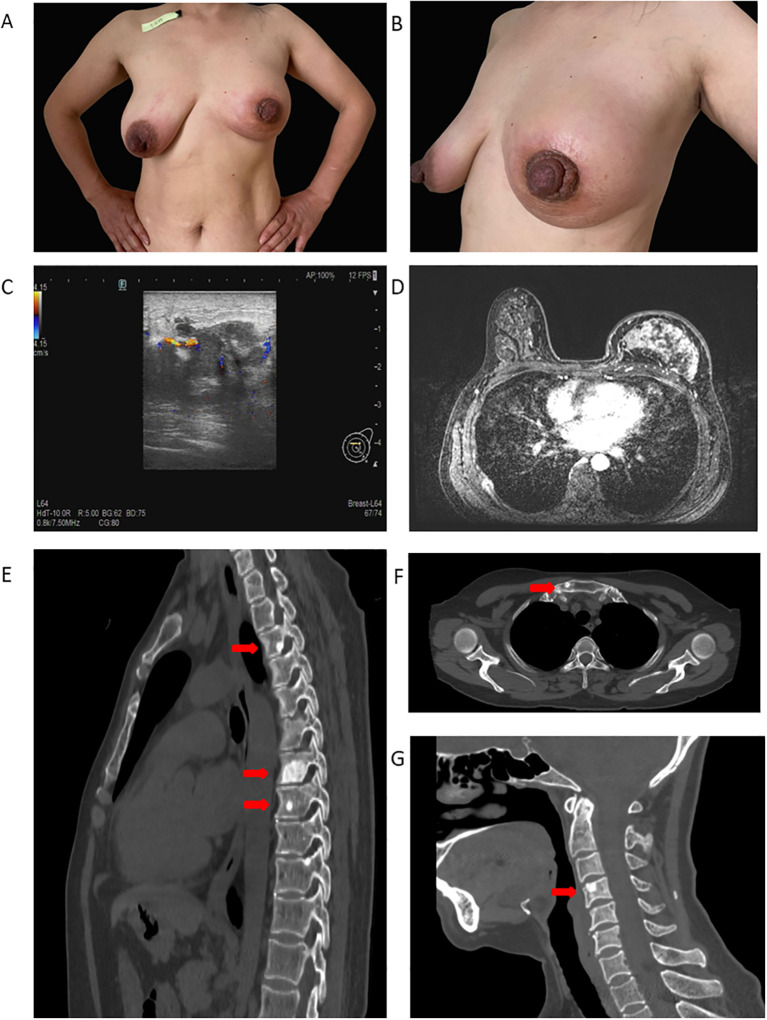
Imaging features at initial presentation. (A, B) Frontal and lateral photographs of the breast at the initial visit. (C) Color Doppler ultrasonography demonstrating diffuse hypoechoic and heterogeneous parenchymal echoes involving nearly the entire left breast. (D) Breast MRI showing diffuse enhancement, skin thickening, and an ill-defined nodular lesion with a type III washout kinetic curve. (E–G) Contrast-enhanced CT showing focal osteolytic destruction involving parts of the thoracic vertebrae, cervical vertebrae, and the manubrium.
